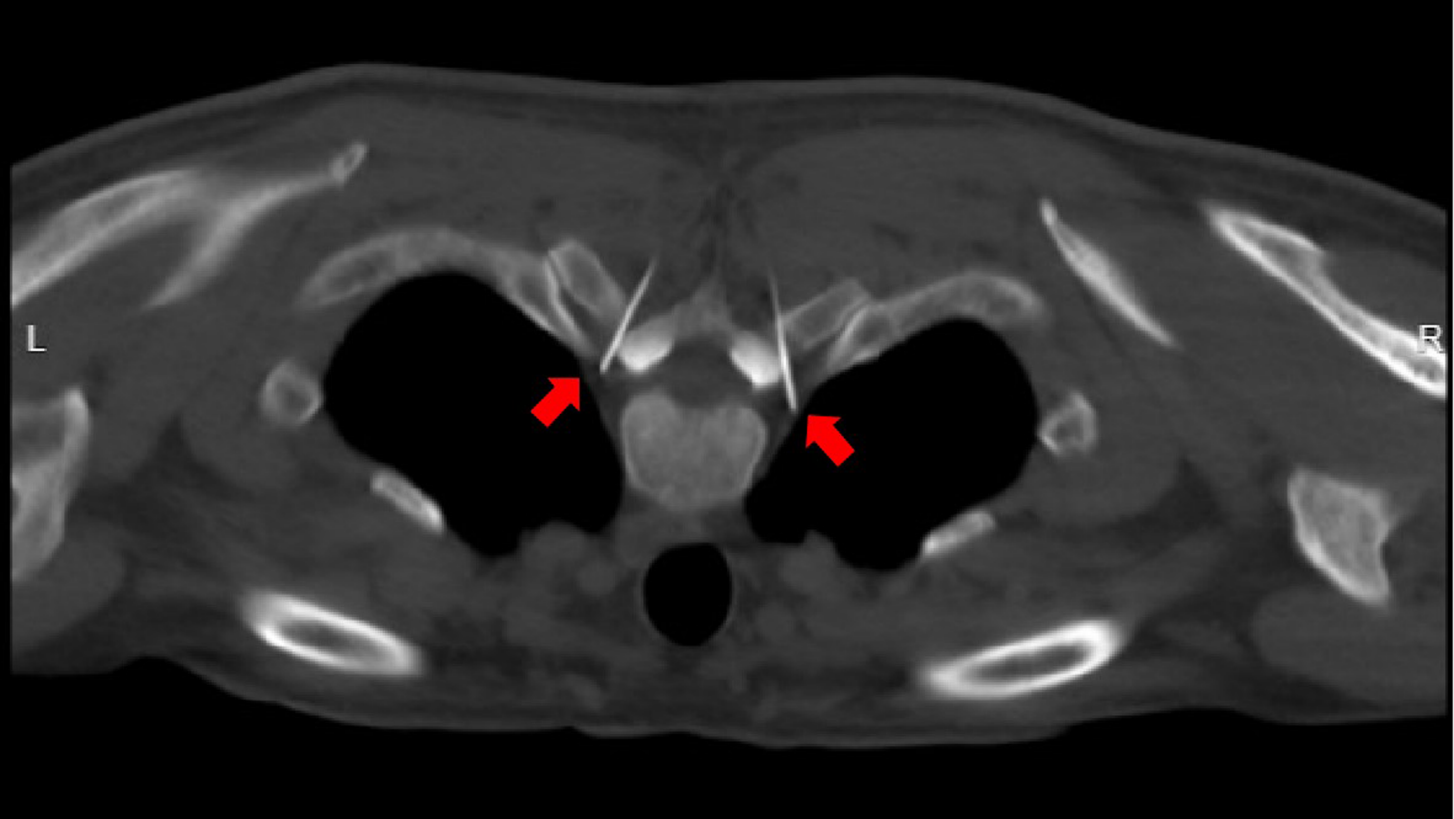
Under CT guidance, the needle tip reaches the predetermined target point (indicated by the red arrow) along the designed puncture path.
